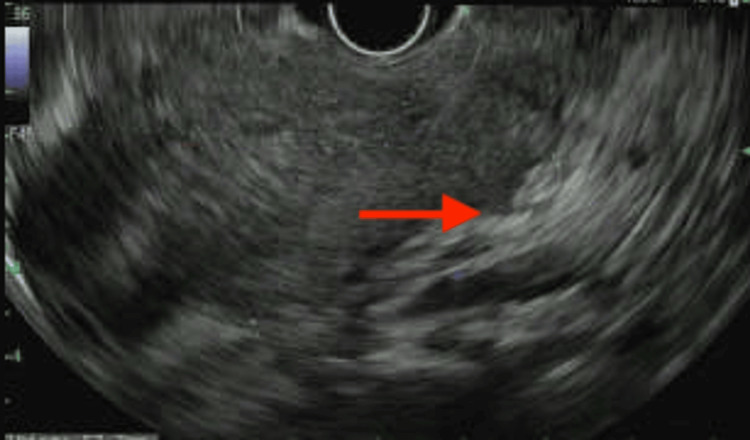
EUS image of the distal rectal mass A large, well-defined hypoechoic lesion measuring 8.5 × 5.1 × 4.0 cm is seen arising from the muscularis propria (red arrow). The hypoechoic mass disrupts the normal layered architecture of the rectal wall. EUS: endoscopic ultrasound
